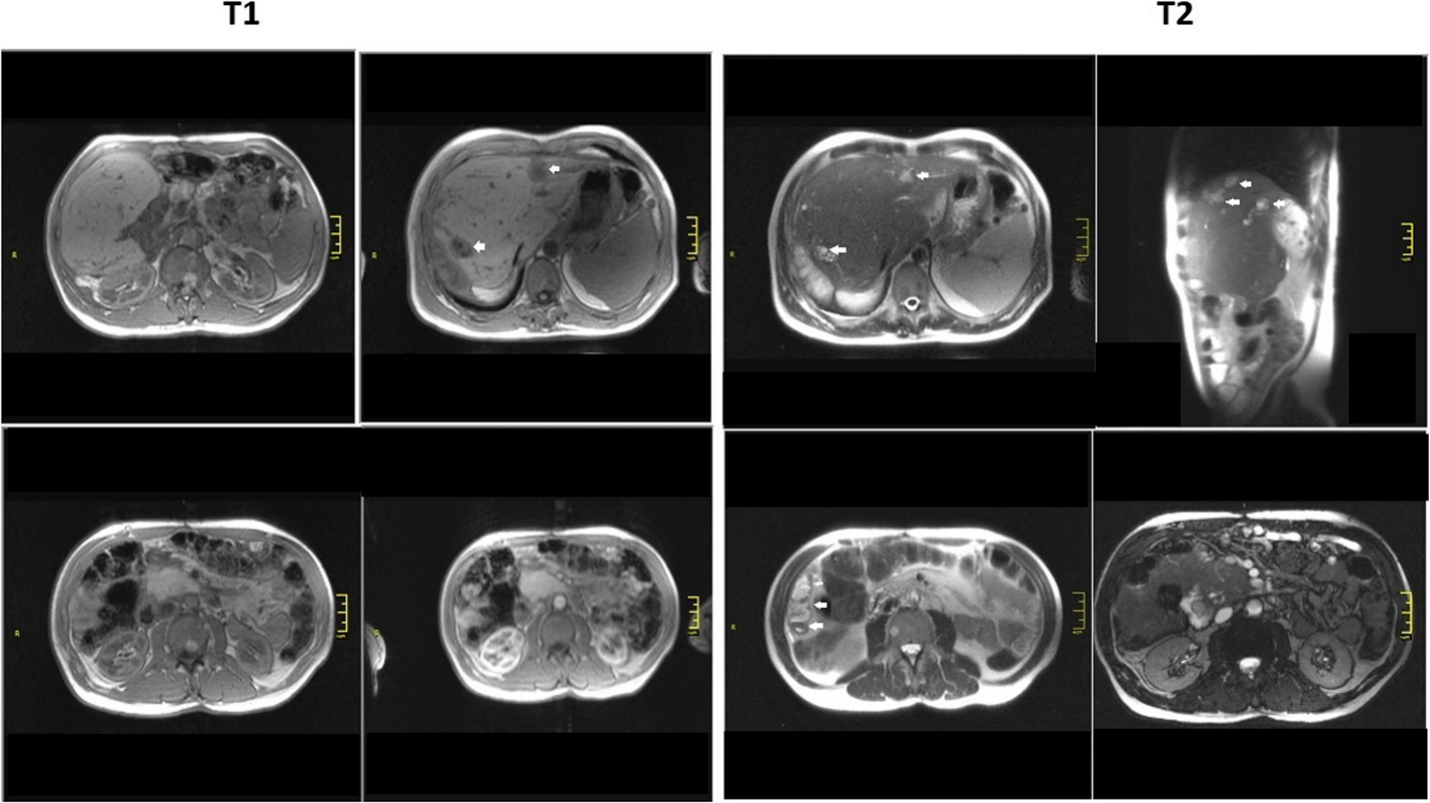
Magnetic resonance imaging from 2011.
